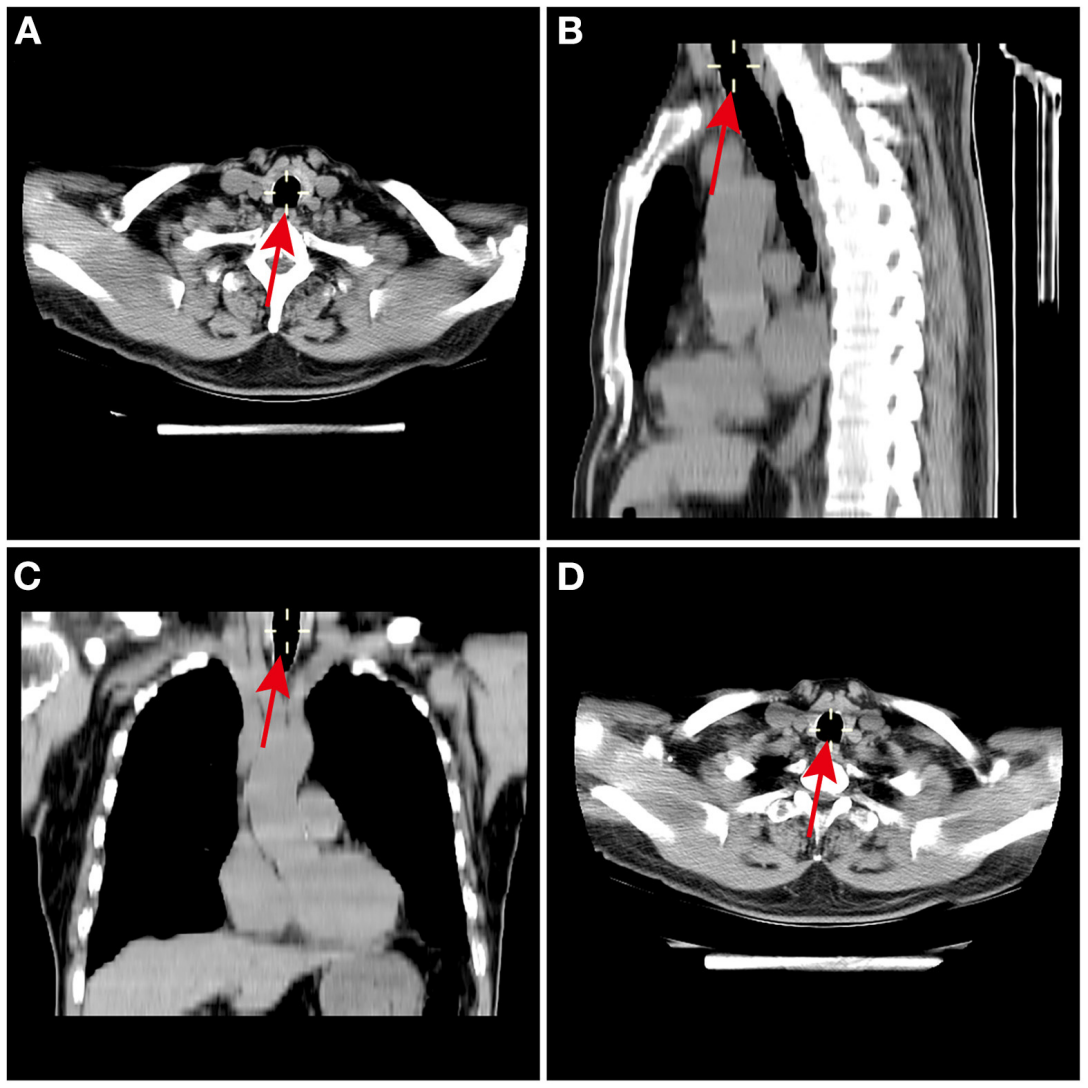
Measurement of the cricoid cartilage using chest HRCT scan MPR. The MPR of the cricoid cartilage was performed using the (A) axial, (B) sagittal, and (C) coronal slices. The declination of TD-C was corrected in 3 dimensions to obtain a strictly orthogonal cut of the cricoid cartilage axis. (D) The TD-C were measured on the MPR at the lower border of the cricoid ring. TD-C, transverse diameter of cricoid cartilage.
